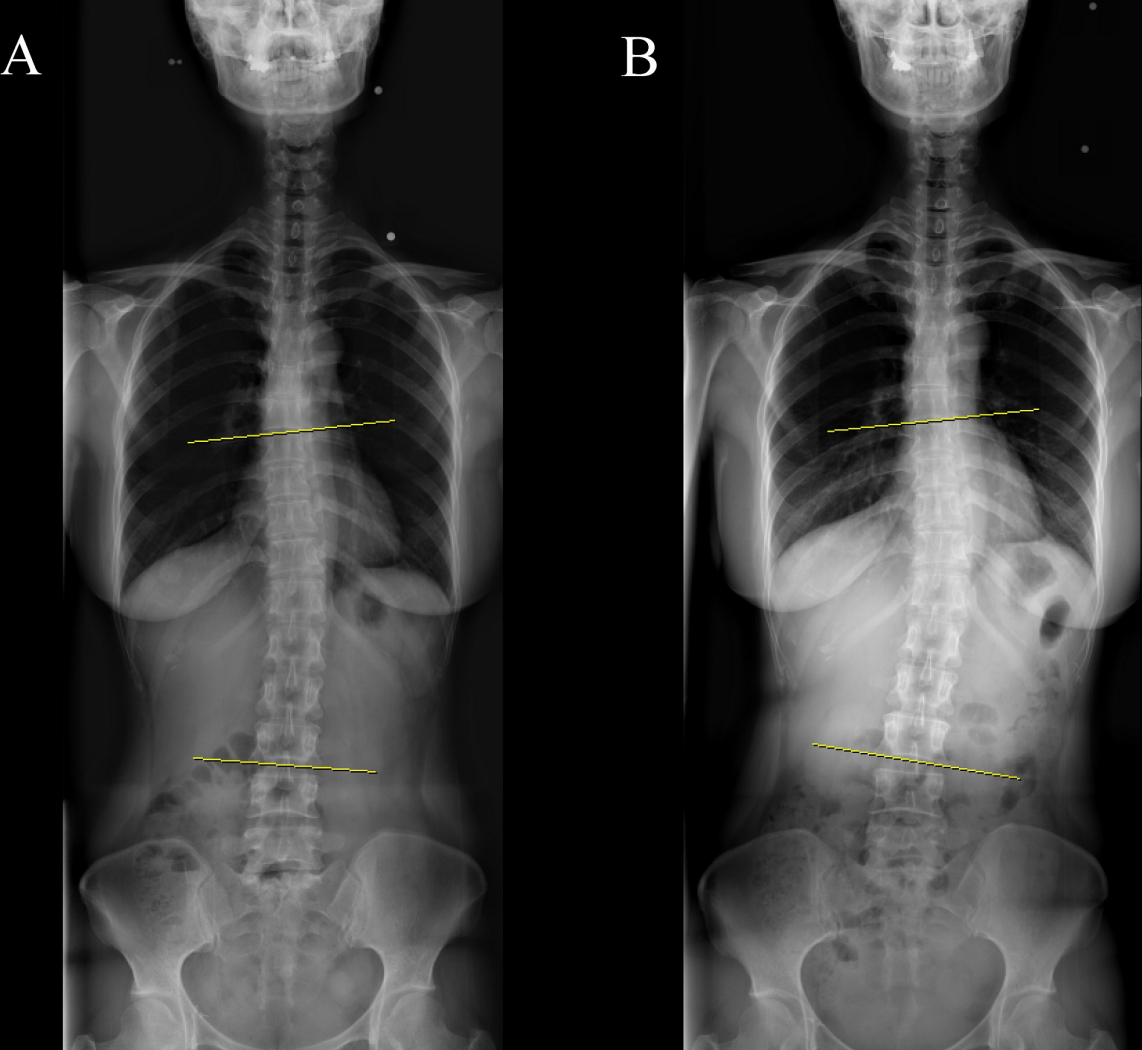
Demonstration of Cobb angle on whole-spine radiograph in one patient with a significant change in spinal curve; (A) Initial radiograph (B) Follow-up radiograph.
